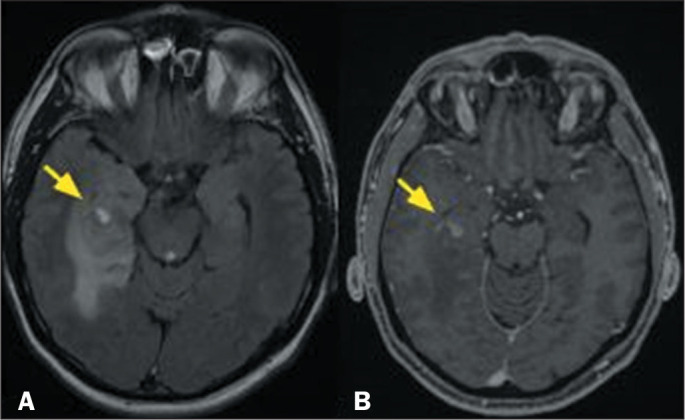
MRI scans of a 53-year-old male patient. A FLAIR sequence (A) showing
